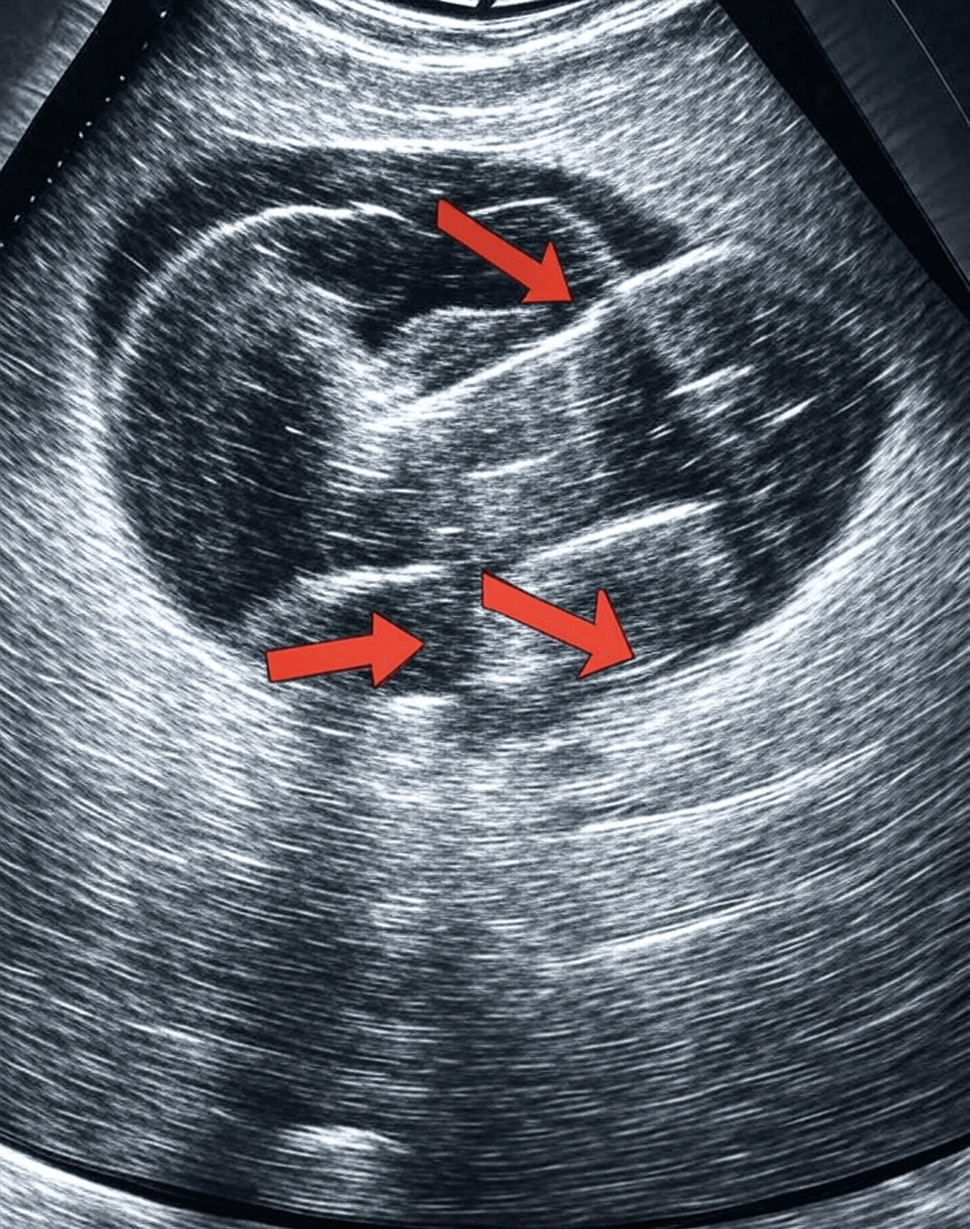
Temporal artery ultrasound showing increased intima-media thickness in the superficial temporal artery The red arrows indicate areas of thickened intima media, consistent with GCA. GCA, giant cell arteritis
